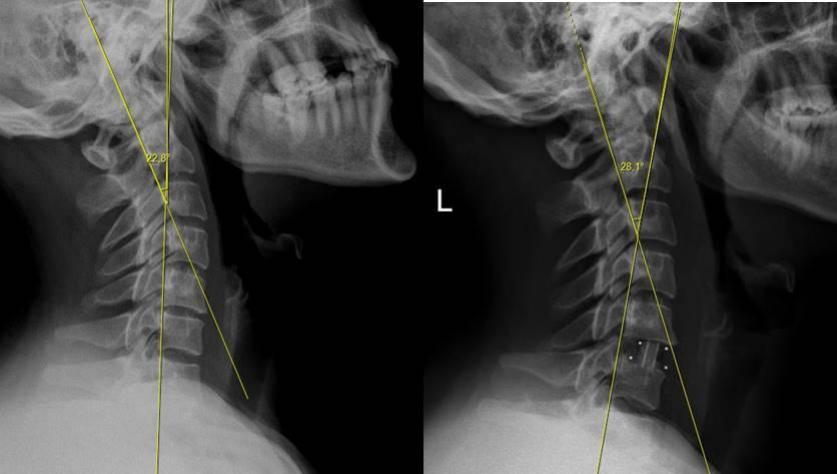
We measured cervical lordosis angle in degrees on lateral X-ray, and we used Baskent University pacs system (Interpacs Version 1.0.0.0, www.interpacs.com) to measure these angles*.
